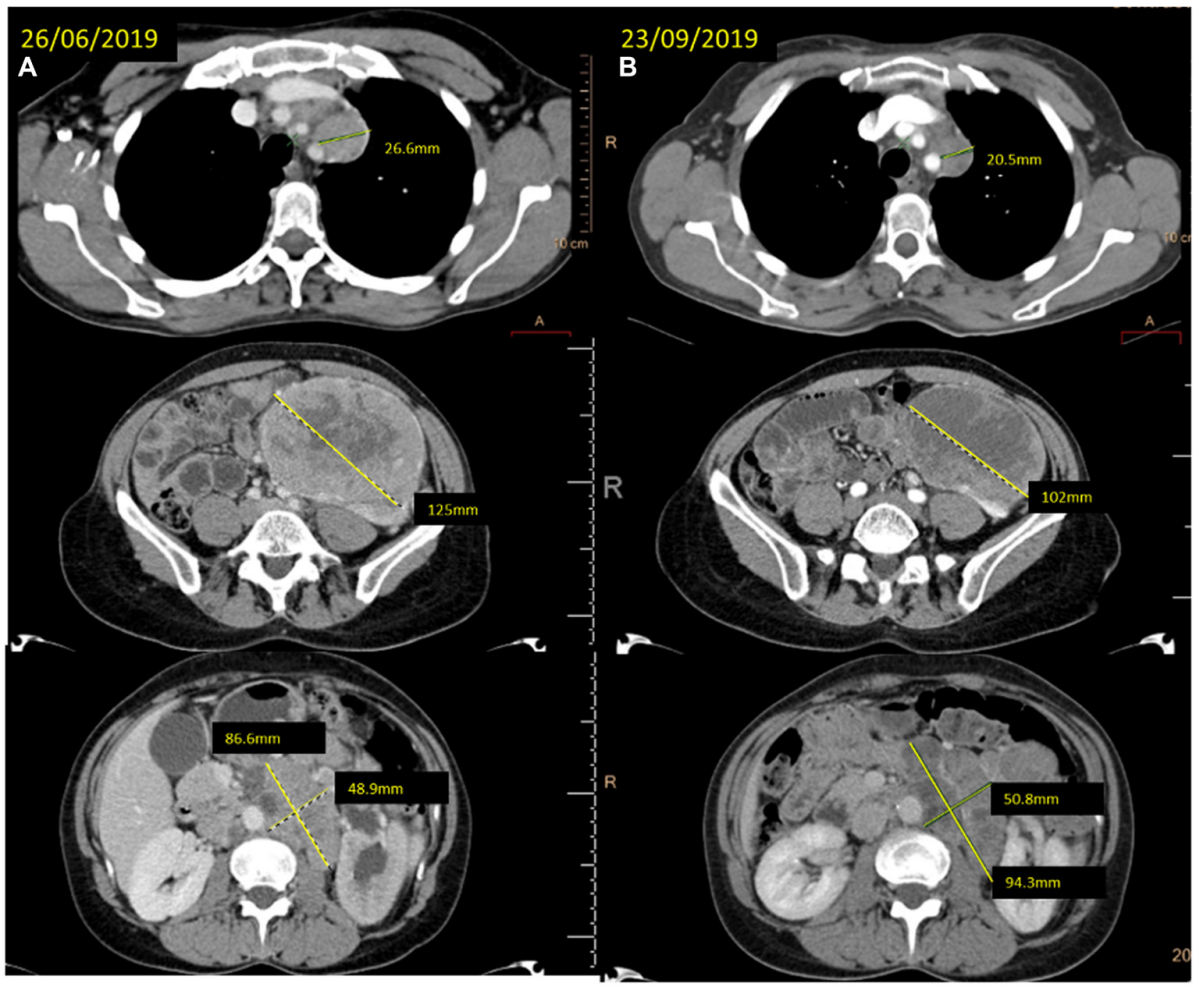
(A) baseline CT scan and (B) the reassessment CT scan showing the response achieved after 12 weeks of treatment with cabozantinib at different levels (mediastinal lymph nodes, left kidney mass and retroperitoneal lymph nodes).
